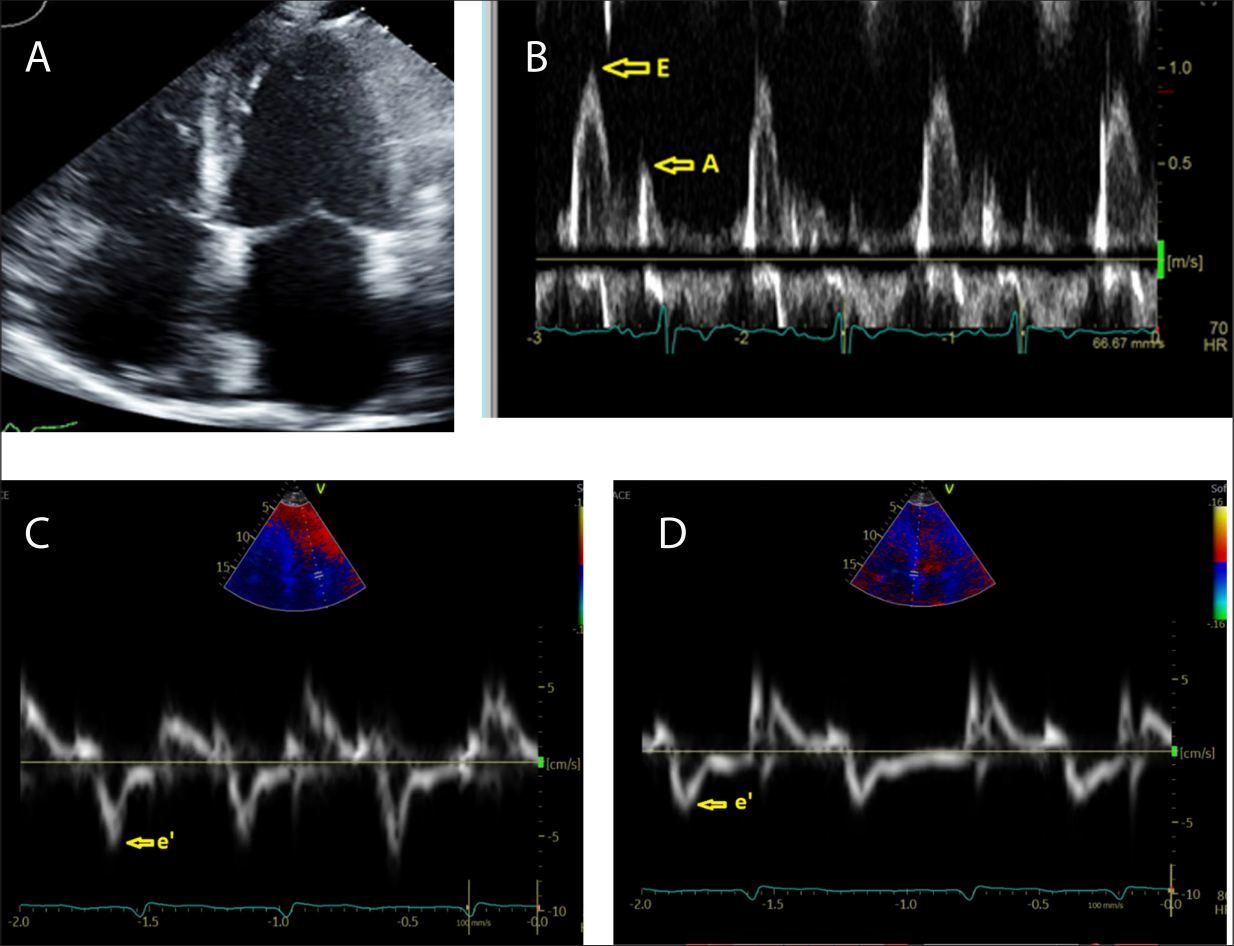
(A) Apical 4-chamber view showing biatrial enlargement. (B) Mitral inflow doppler showing increased E/A ratio (feature of diastolic dysfunction). (C, D) Lateral and septal mitral annular tissue doppler showing decreased e’ velocity.
